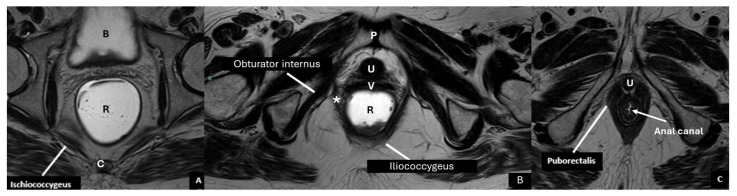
Pelvic muscles on axial MRI. Axial T2-weighted images (A–C) demonstrate normal pelvic diaphragm anatomy, including the iliococcygeus, ischiococcygeus, puborectalis (*), and obturator internus muscles. C = coccyx, P = pubic symphysis, R = rectum, U = urethra, B = bladder, V = vagina.
